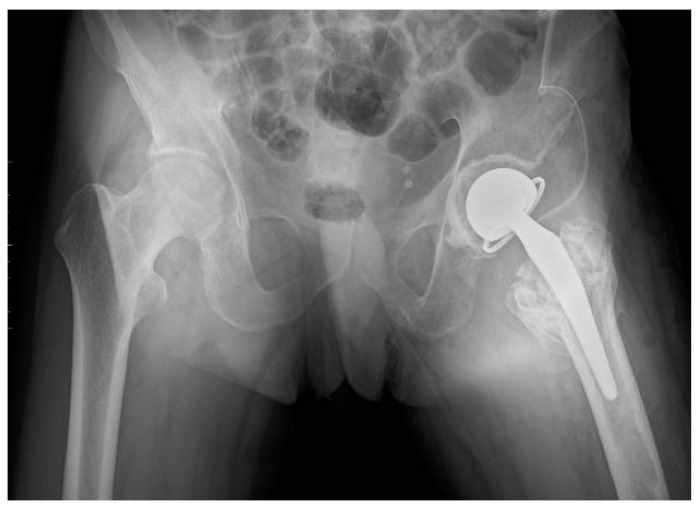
X-ray after surgery. Implantation of a total snap-on endoprosthesis of the left hip joint. AP view.
